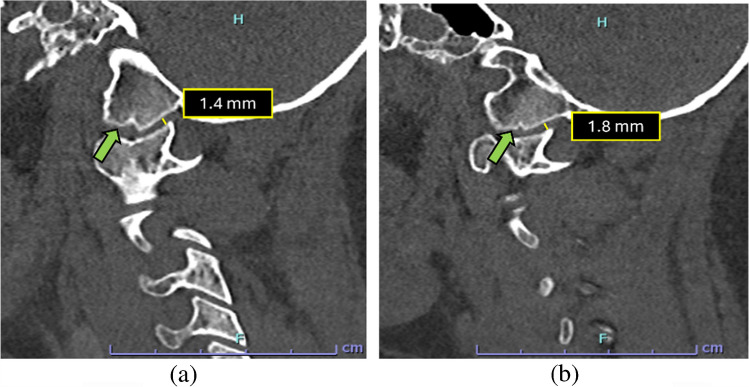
Parasagittal CT images of the cervical spine: right (a) and left (b) demonstrating normal occipital condyle–C1 interval (CCI) measurements. The minimum joint space at the lateral-most aspect of the joint in the parasagittal section (normal <2.5 mm) is measured, with care taken to exclude the medial occipital notch (*green arrow*) to avoid false-positive interpretation. The medial occipital notch is shown on either side by the *green arrow*. The morphology of the occipital notch is different on either side and this is a common finding
